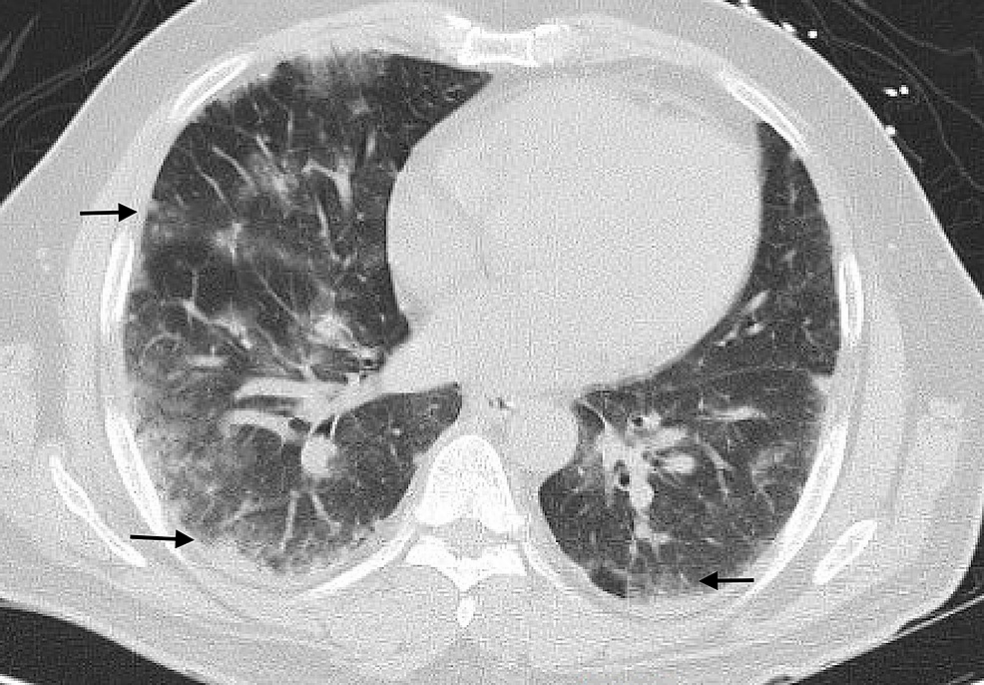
Cross-sectional view of CT chest showing bilateral involvement of ground glass opacities predominately in the periphery (black arrows). CT, computed tomography
